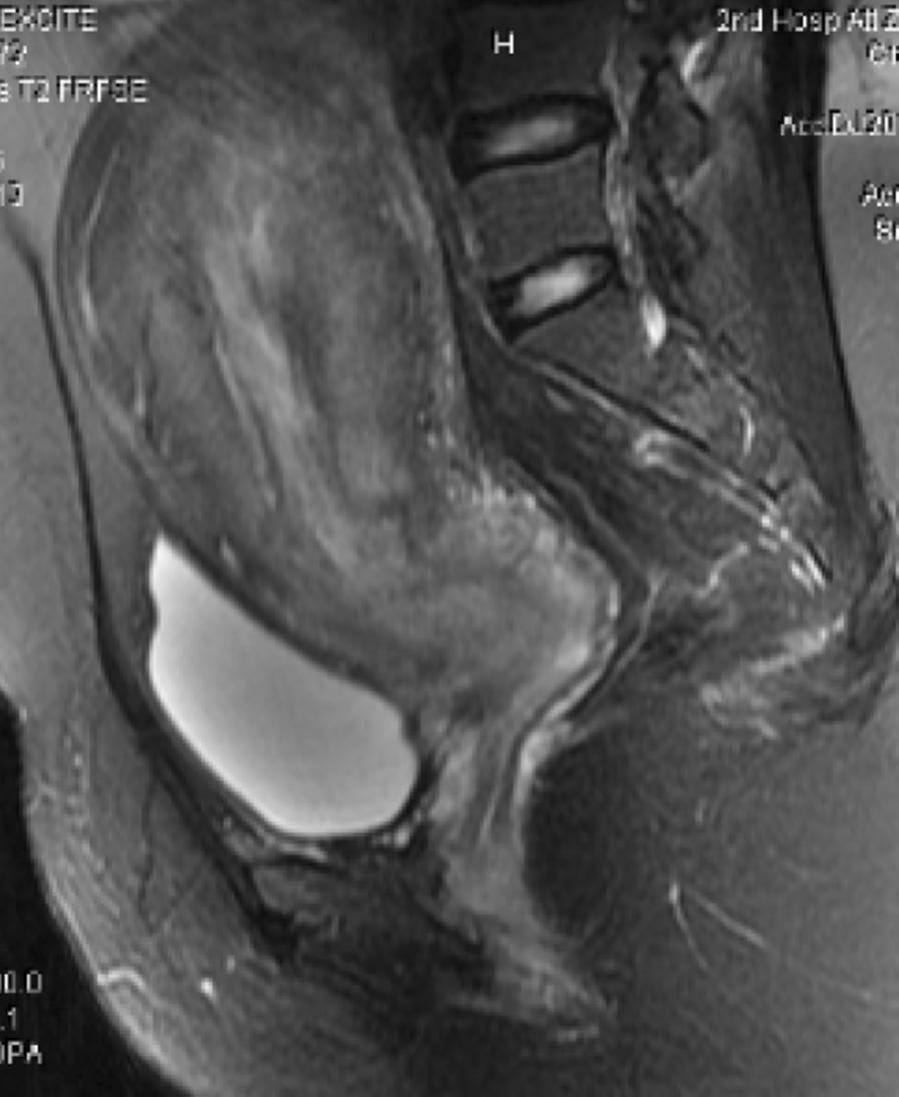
MRI demonstrates segment of placenta extending outside of the visible myometrium
